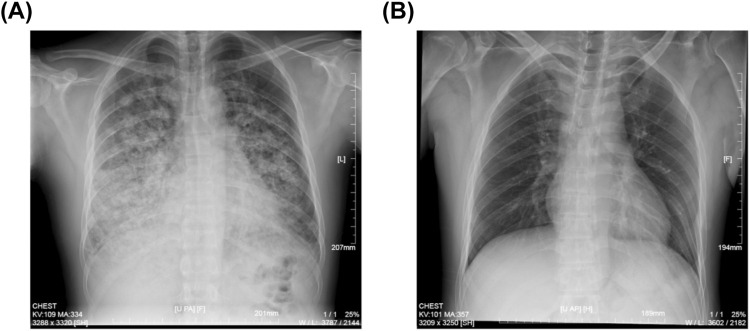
Chest X-ray images of the patient. (A) Spine position of chest X-ray image showing the HAPE at the patient’s admission to our hospital. (B) Upright position of chest X-ray image after continuous oxygen supplementation of 3 days.
